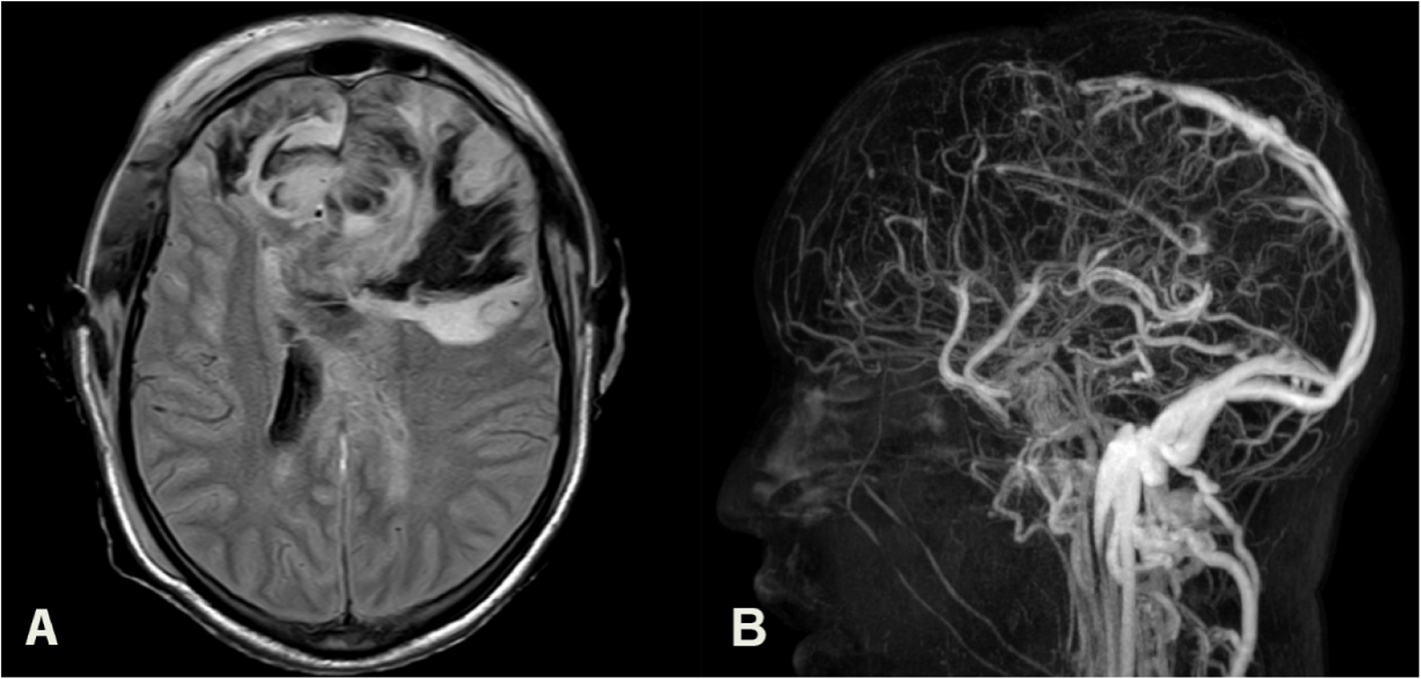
Case 2 Neuroimaging. A: Axial MR image -bilateral frontal lobe extensive haemorrhage with intraventricular extension. B: MR Venogram showing filling defects in the anterior half of the superior sagittal sinus
